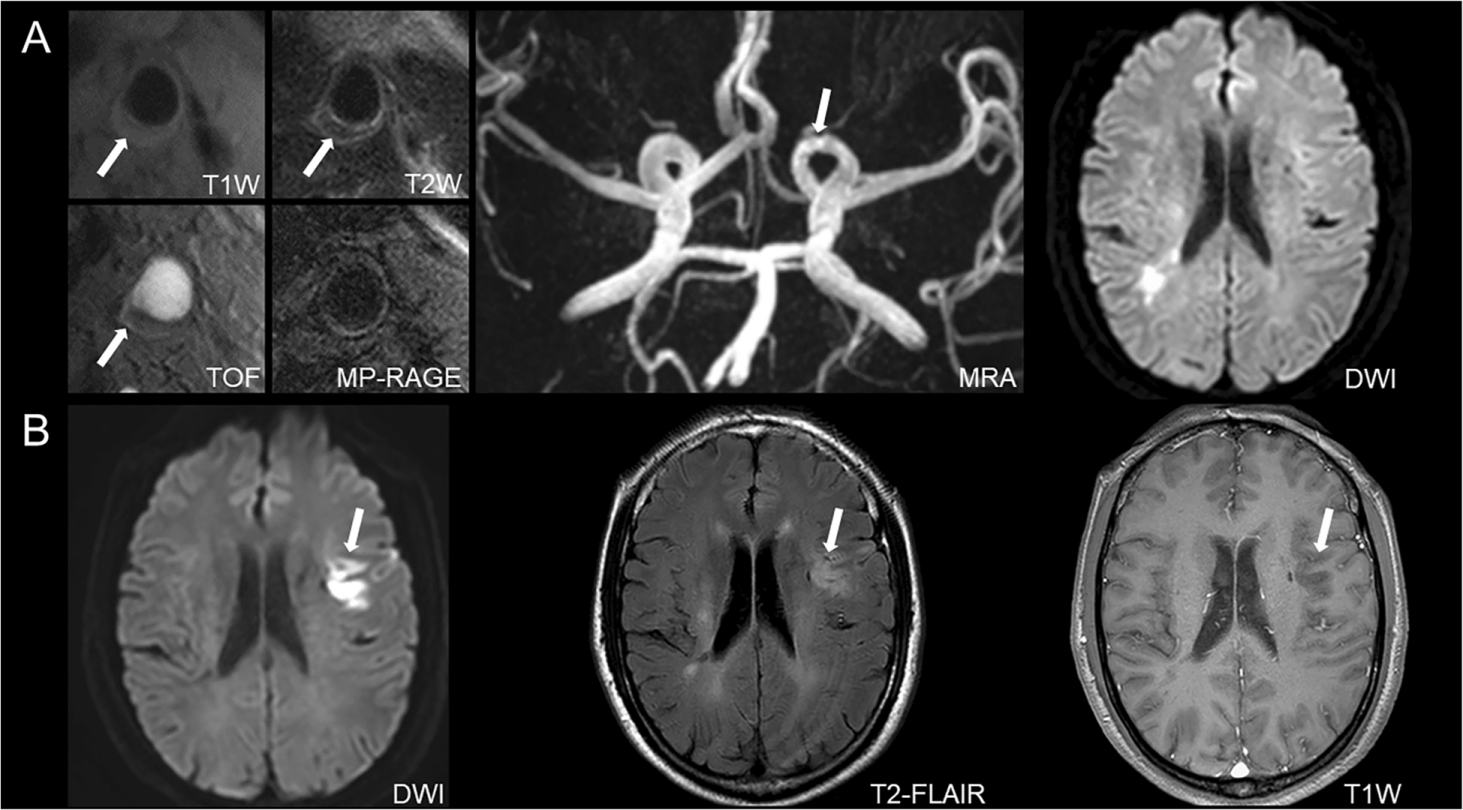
Patient with co-existing intracranial artery stenosis and extracranial carotid LRNC developed recurrent stroke. An example for a 63 years old male patient who had atherosclerotic plaque with lipid-rich necrotic core in the left extracranial carotid artery bifurcation (white arrows on A [T1 weighted (T1W), T2 weighted (T2w) and time-of-flight (TOF)]) and intracranial artery stenosis (white arrow on A [MRA]). After a year of follow-up, a new acute infarction was detected in the left hemisphere (white arrows on B [diffusion weighted imaging (DWI), T2-FLAIR and T1w])
